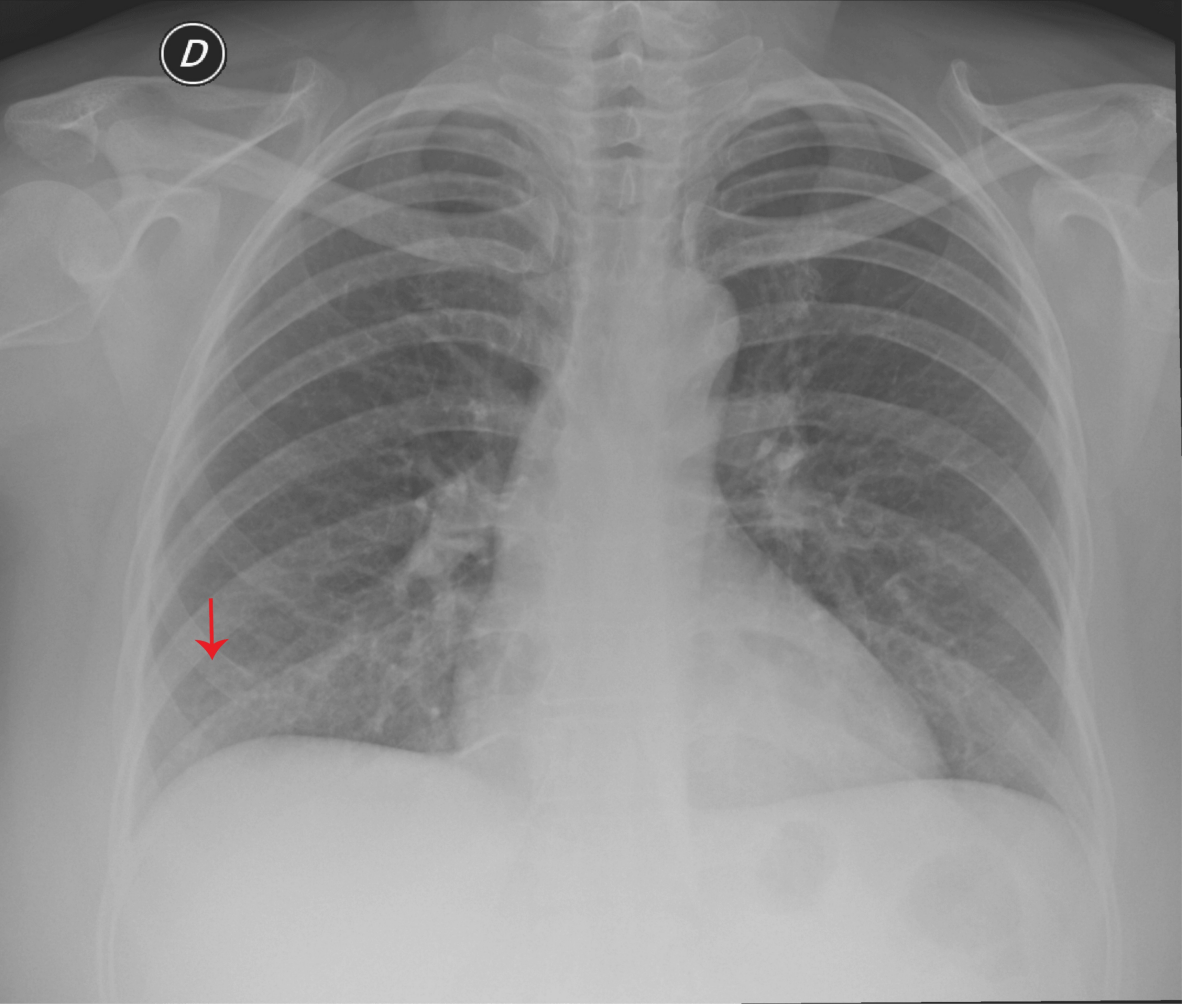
Chest X-ray The right costophrenic angle appears blunted (red arrow), indicating a possible mild pleural effusion.
